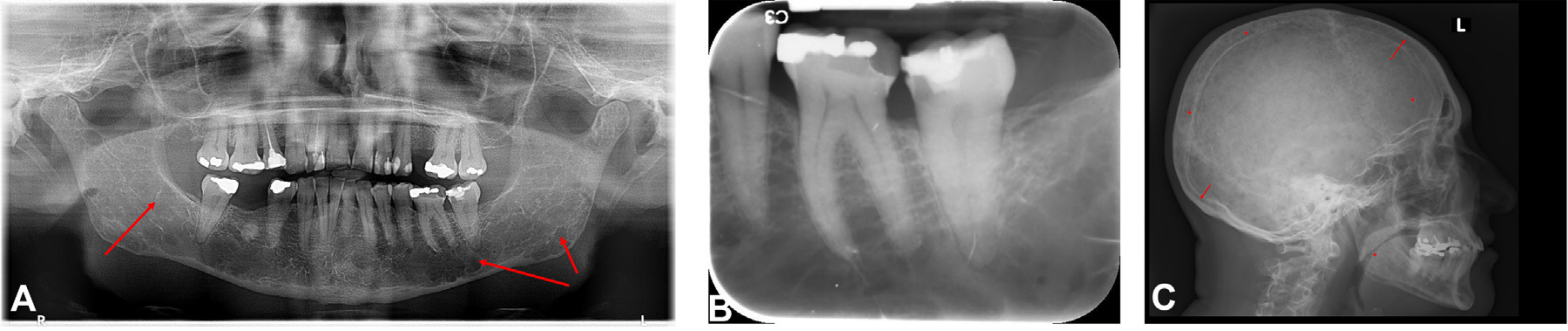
Case 1: (A) Panoramic radiograph showing multiple, well-defined lucencies located bilaterally, extending from the angles of the mandible to the ascending rami (red arrows). Both inferior alveolar nerve canals lack cortication. (B) Periapical radiograph of #36 and 37 showing compromised restorations. (C) Lateral skull view showing multiple small, lucent lesions involving the entire skull and mandible (red asterisk), demonstrating endosteal scalloping (red arrows) and lytic areas.
